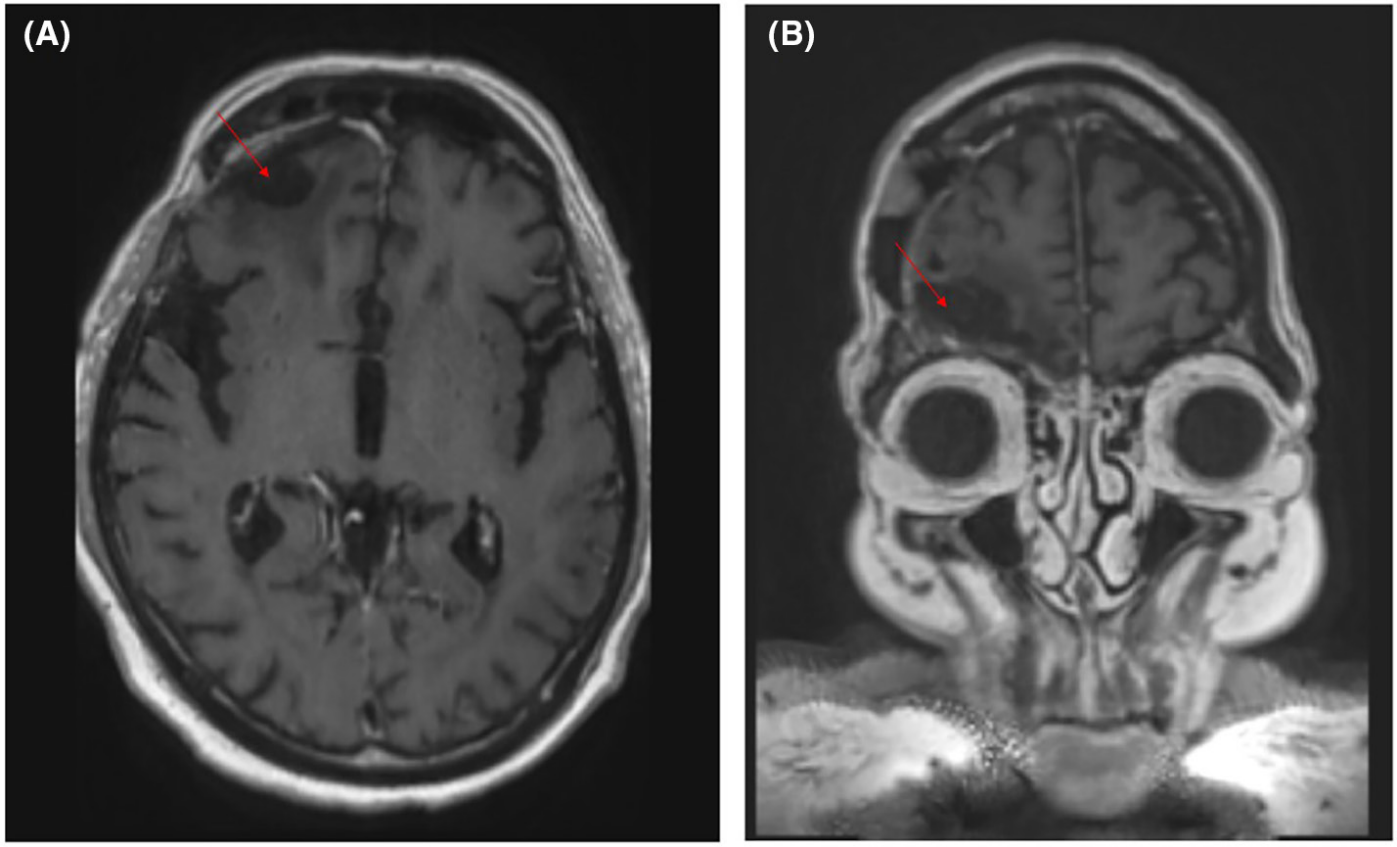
January 18, 2019 (2 years post–chemotherapy and radiation). MRI head, post gadolinium 3D T1 axial and sagittal views showing no evidence of recurrence in the brain.
